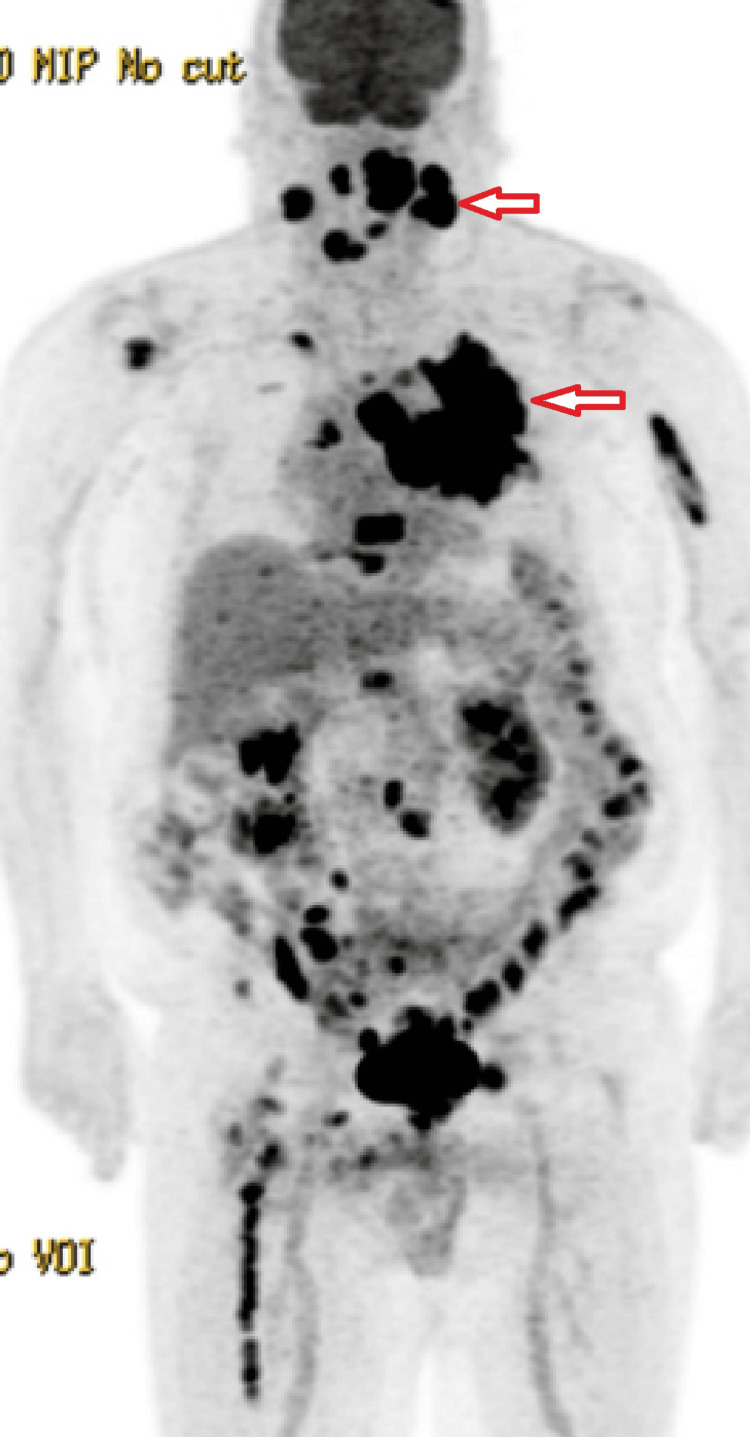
CT/PET at diagnosis with tonsillar mass and lung mass
